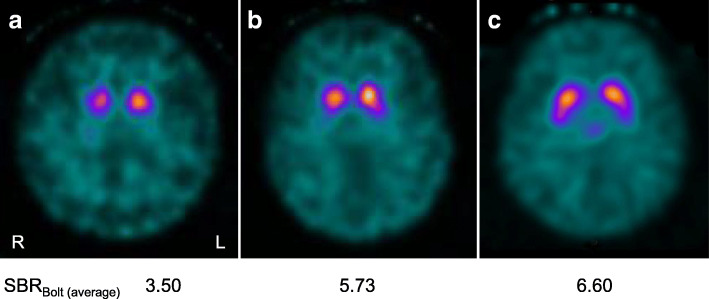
Dopamine transporter single-photon emission computed tomography using ^123^I-ioflupane. The proband (II-1) in Family 1(a) and an unrelated 60-year-old patient with Parkinson’s disease (b) both showed decreased bilateral striatal uptake of ^123^I-ioflupane, more marked in the putamen, while another unrelated 65-year-old patient with drug-induced parkinsonism (c) showed a normal pattern of uptake. The age-matched values for specific binding ratio (SBR) refer to 7.50 ± 1.35 (mean ± SD)
